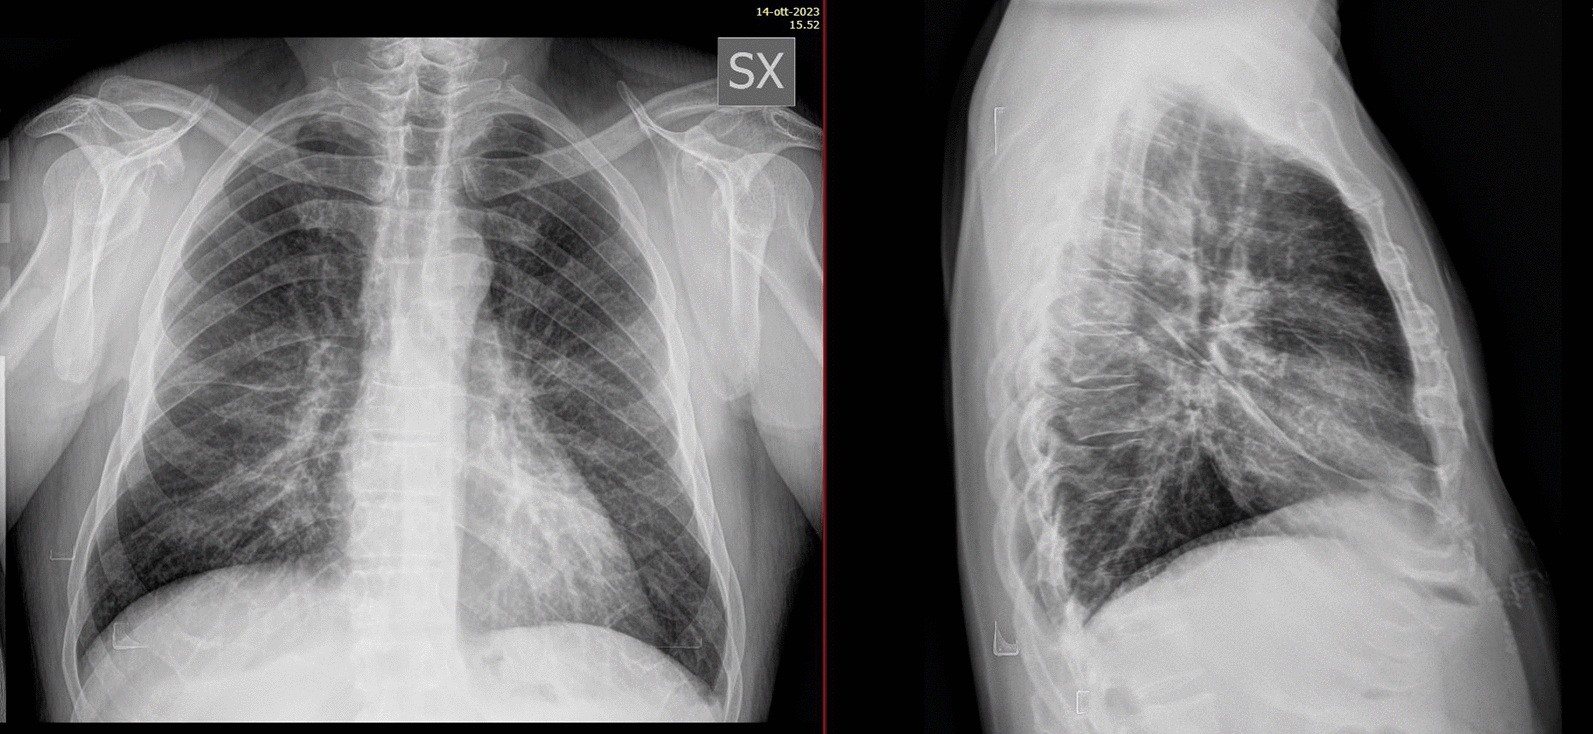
Chest X-ray during at hospital admission
